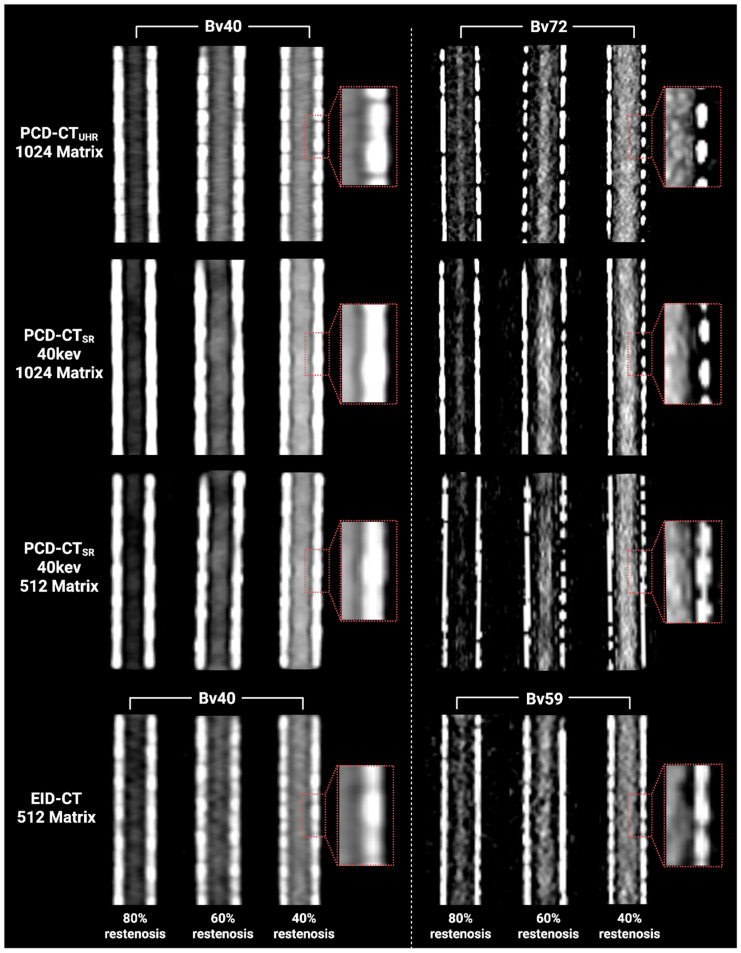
Visualization of in-stent restenosis across different CT scanners and reconstruction kernels. Sharper kernels showed better restenosis visibility on both CT systems. PCD-CT_SR-Bv72-40keV_ further enhanced the restenosis visibility, especially for 40% and 80% restenosis. The red dashed boxes indicate magnified views of the stent strut.
